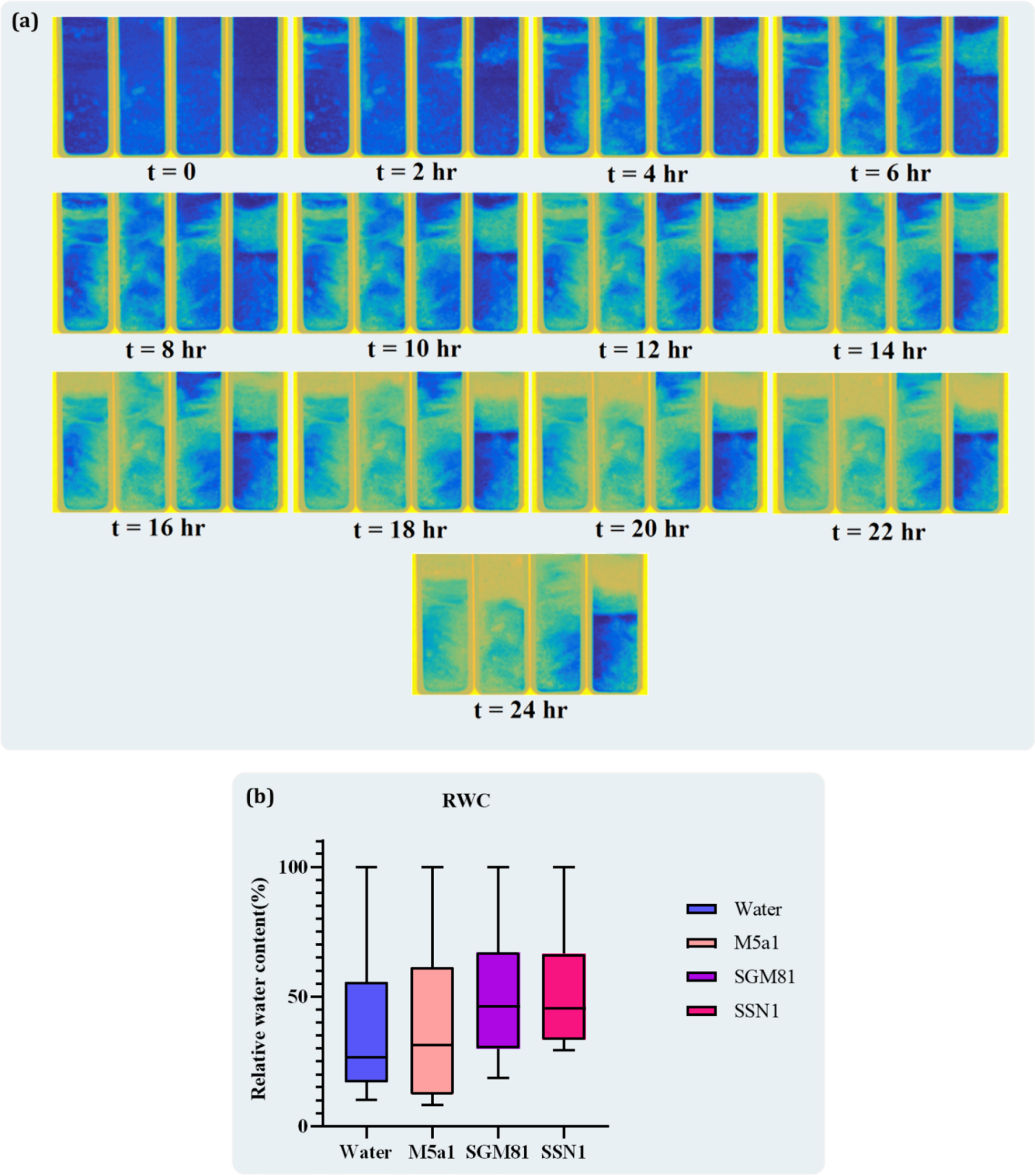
(a) Drying-out progression—Neutron radiographs at different time intervals. The samples are arranged as (from left to right) control (water), M5a1, SGM81, and SSN1 and (b) RWC of all the samples recorded during neutron radiography at an interval of every four hours. The RWC of M5a1 was almost similar to water, whereas SGM81 and SSN1 were showing maximum RWC ratio.
